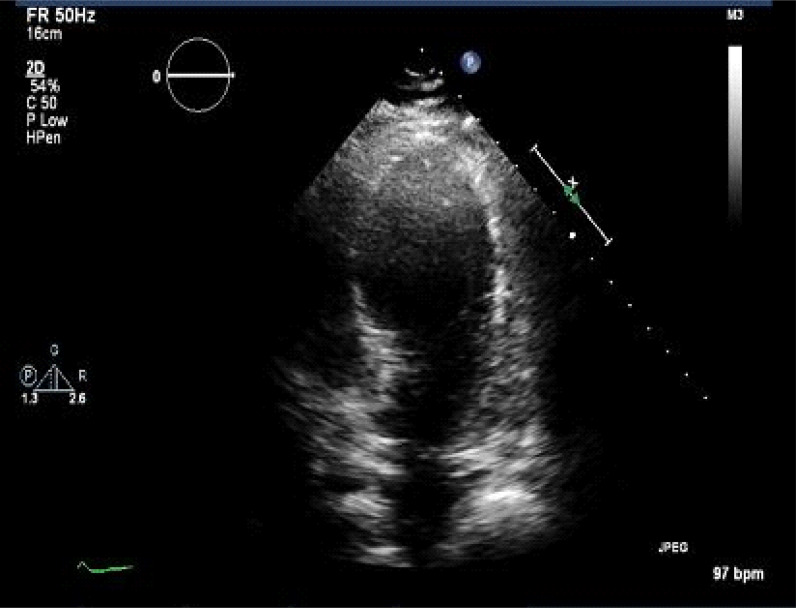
Echocardiography showed septal, lateral mid-chamber, and apical hypokinesis with dilatation.
